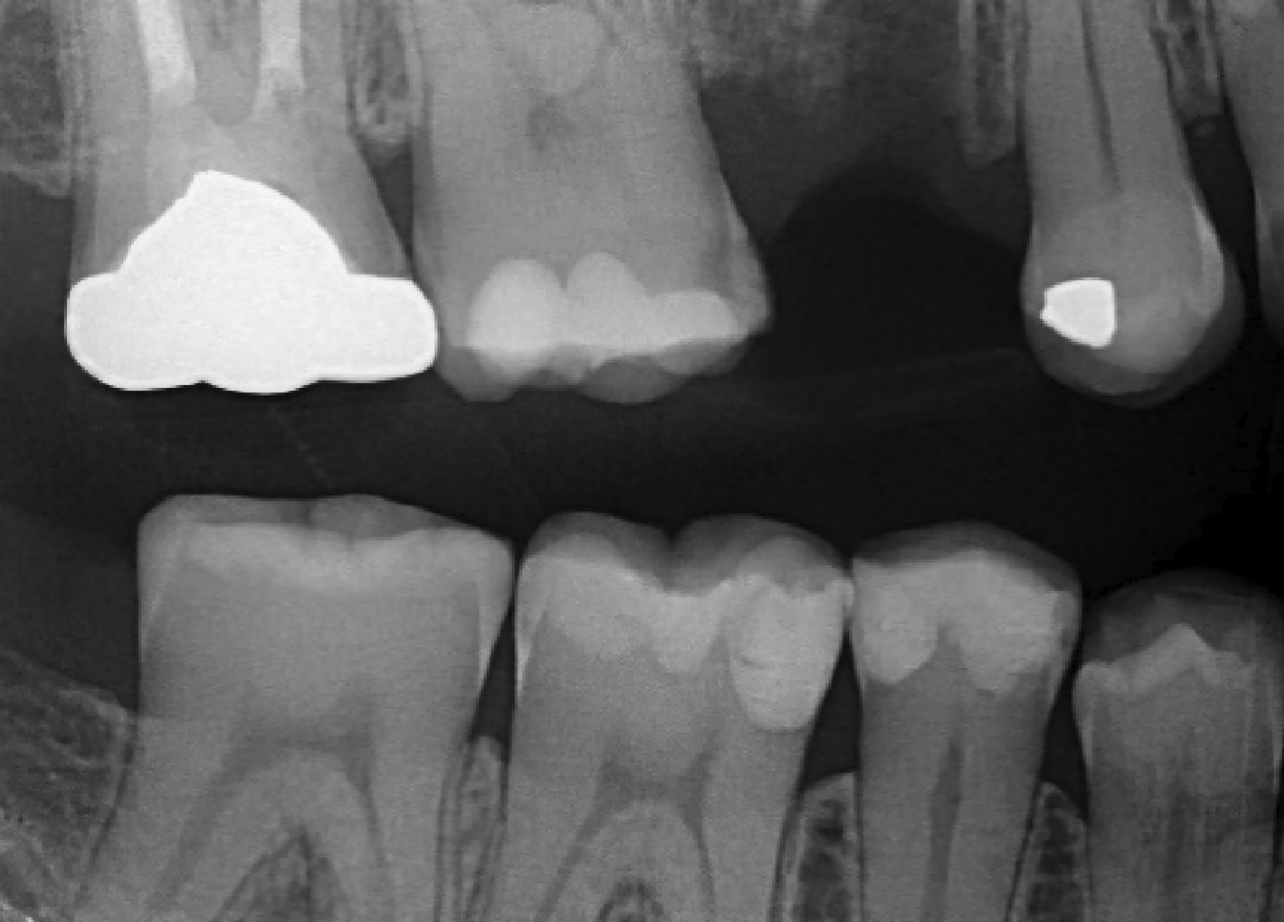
Bitewing radiograph at 5‐year follow‐up.
